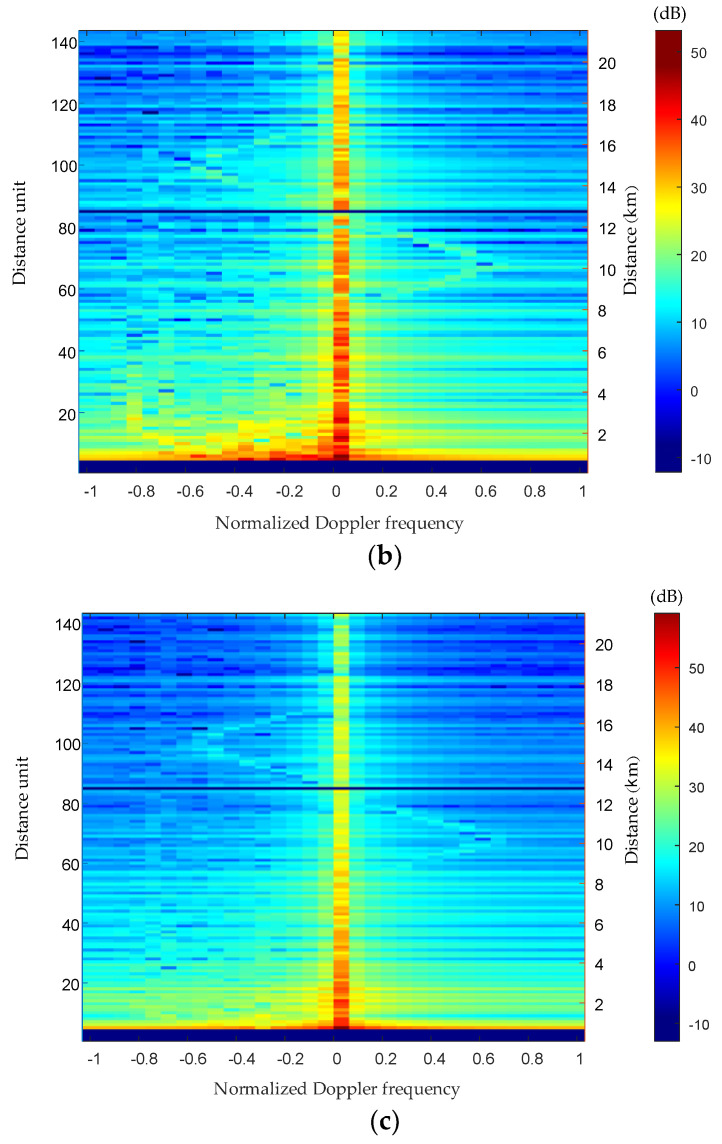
Comparison of Distance-Doppler spectra before and after compensation of fuselage frustum conformal array. (a) before compensation (b) after DW compensation (c) this paper.
